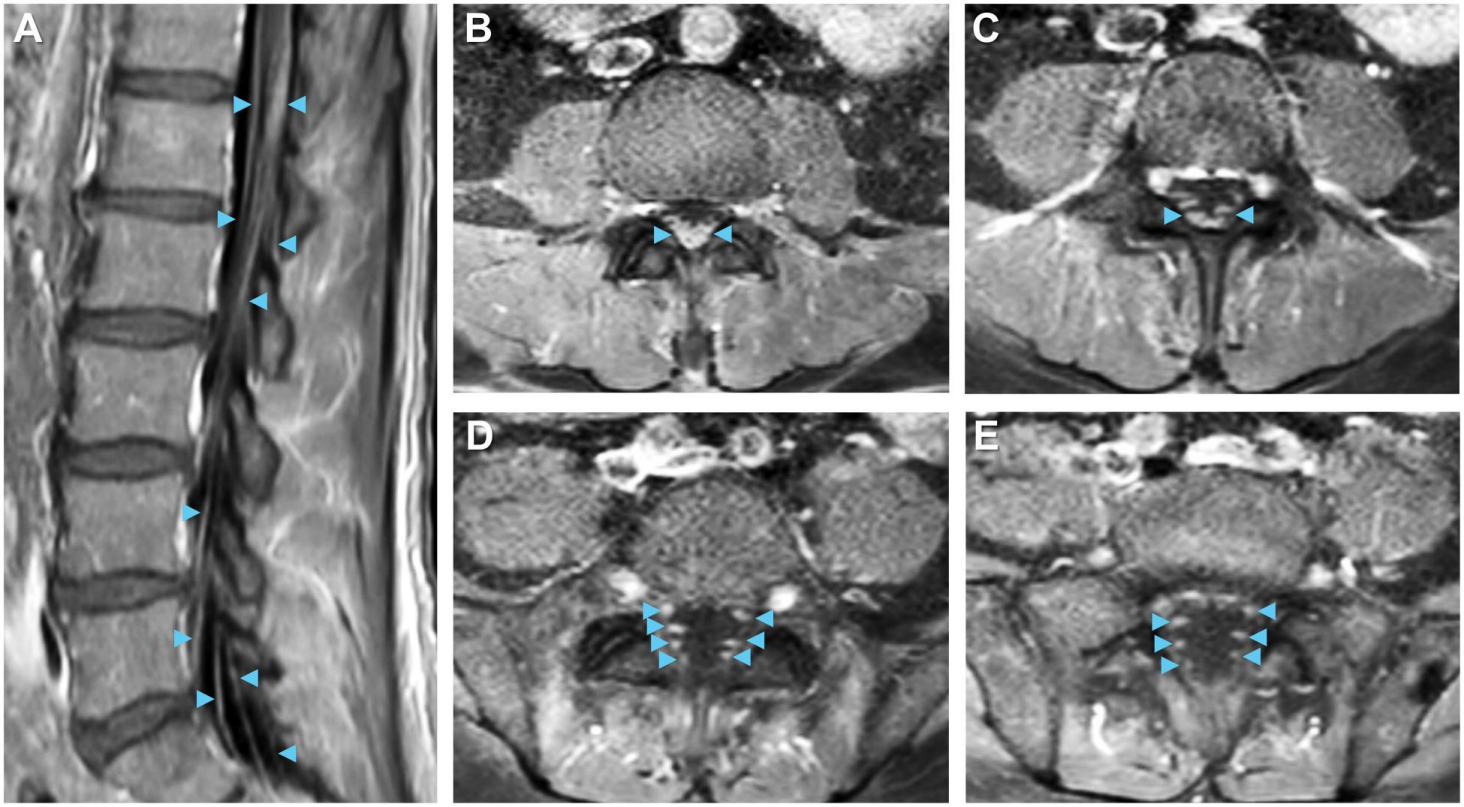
Neuroimaging visualizes lumbar polyradiculitis. Enhancement of bilateral cauda equina nerve roots (arrowheads) near L1-L5 on (A) sagittal and (B-E) descending axial slices supports the diagnosis of lumbar polyradiculitis on T1 post-contrast MRI.
